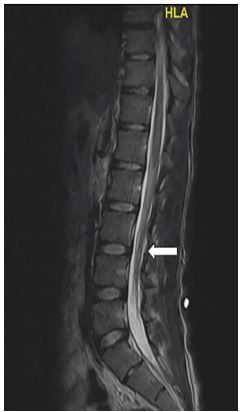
T2-weighted magnetic resonance imaging of the spine. Lower spinal sagittal windows demonstrate diffuse enhancement of the cauda equina (white arrow).
